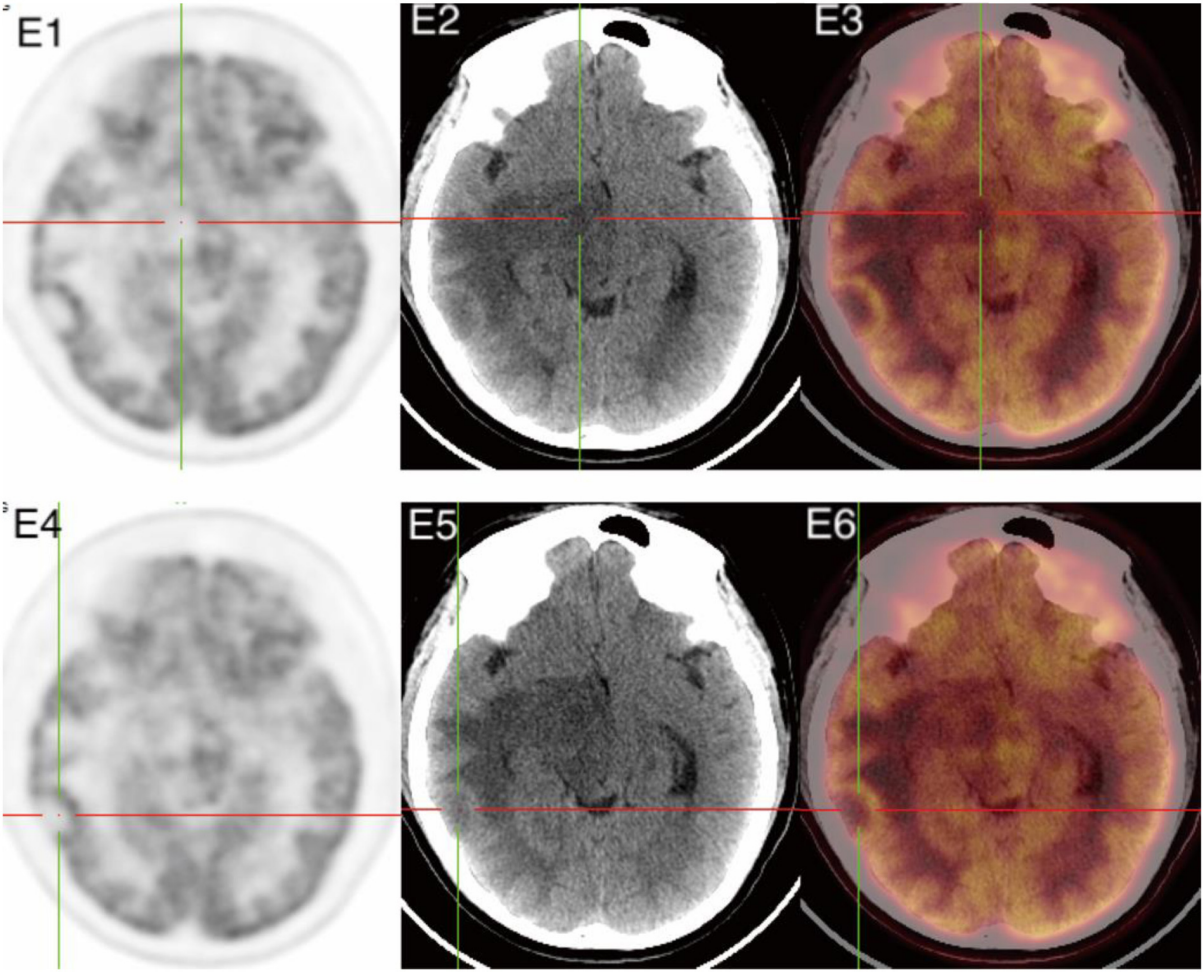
18F-FDG PET/CT images for patient 3. (E1-E3) Reduced radiotracer uptake in the right cerebral peduncle lesion on the FDG PET (E1) image, CT image (E2), and PET/CT fusion image (E3) at the intersection of the red and green lines. (E4-E6) Decreased central radiotracer uptake and slightly increased peripheral uptake in the right temporal lobe lesion on the FDG PET image (E4), CT image (E5), and PET/CT fusion image (E6) at the intersection of the red and green lines.
